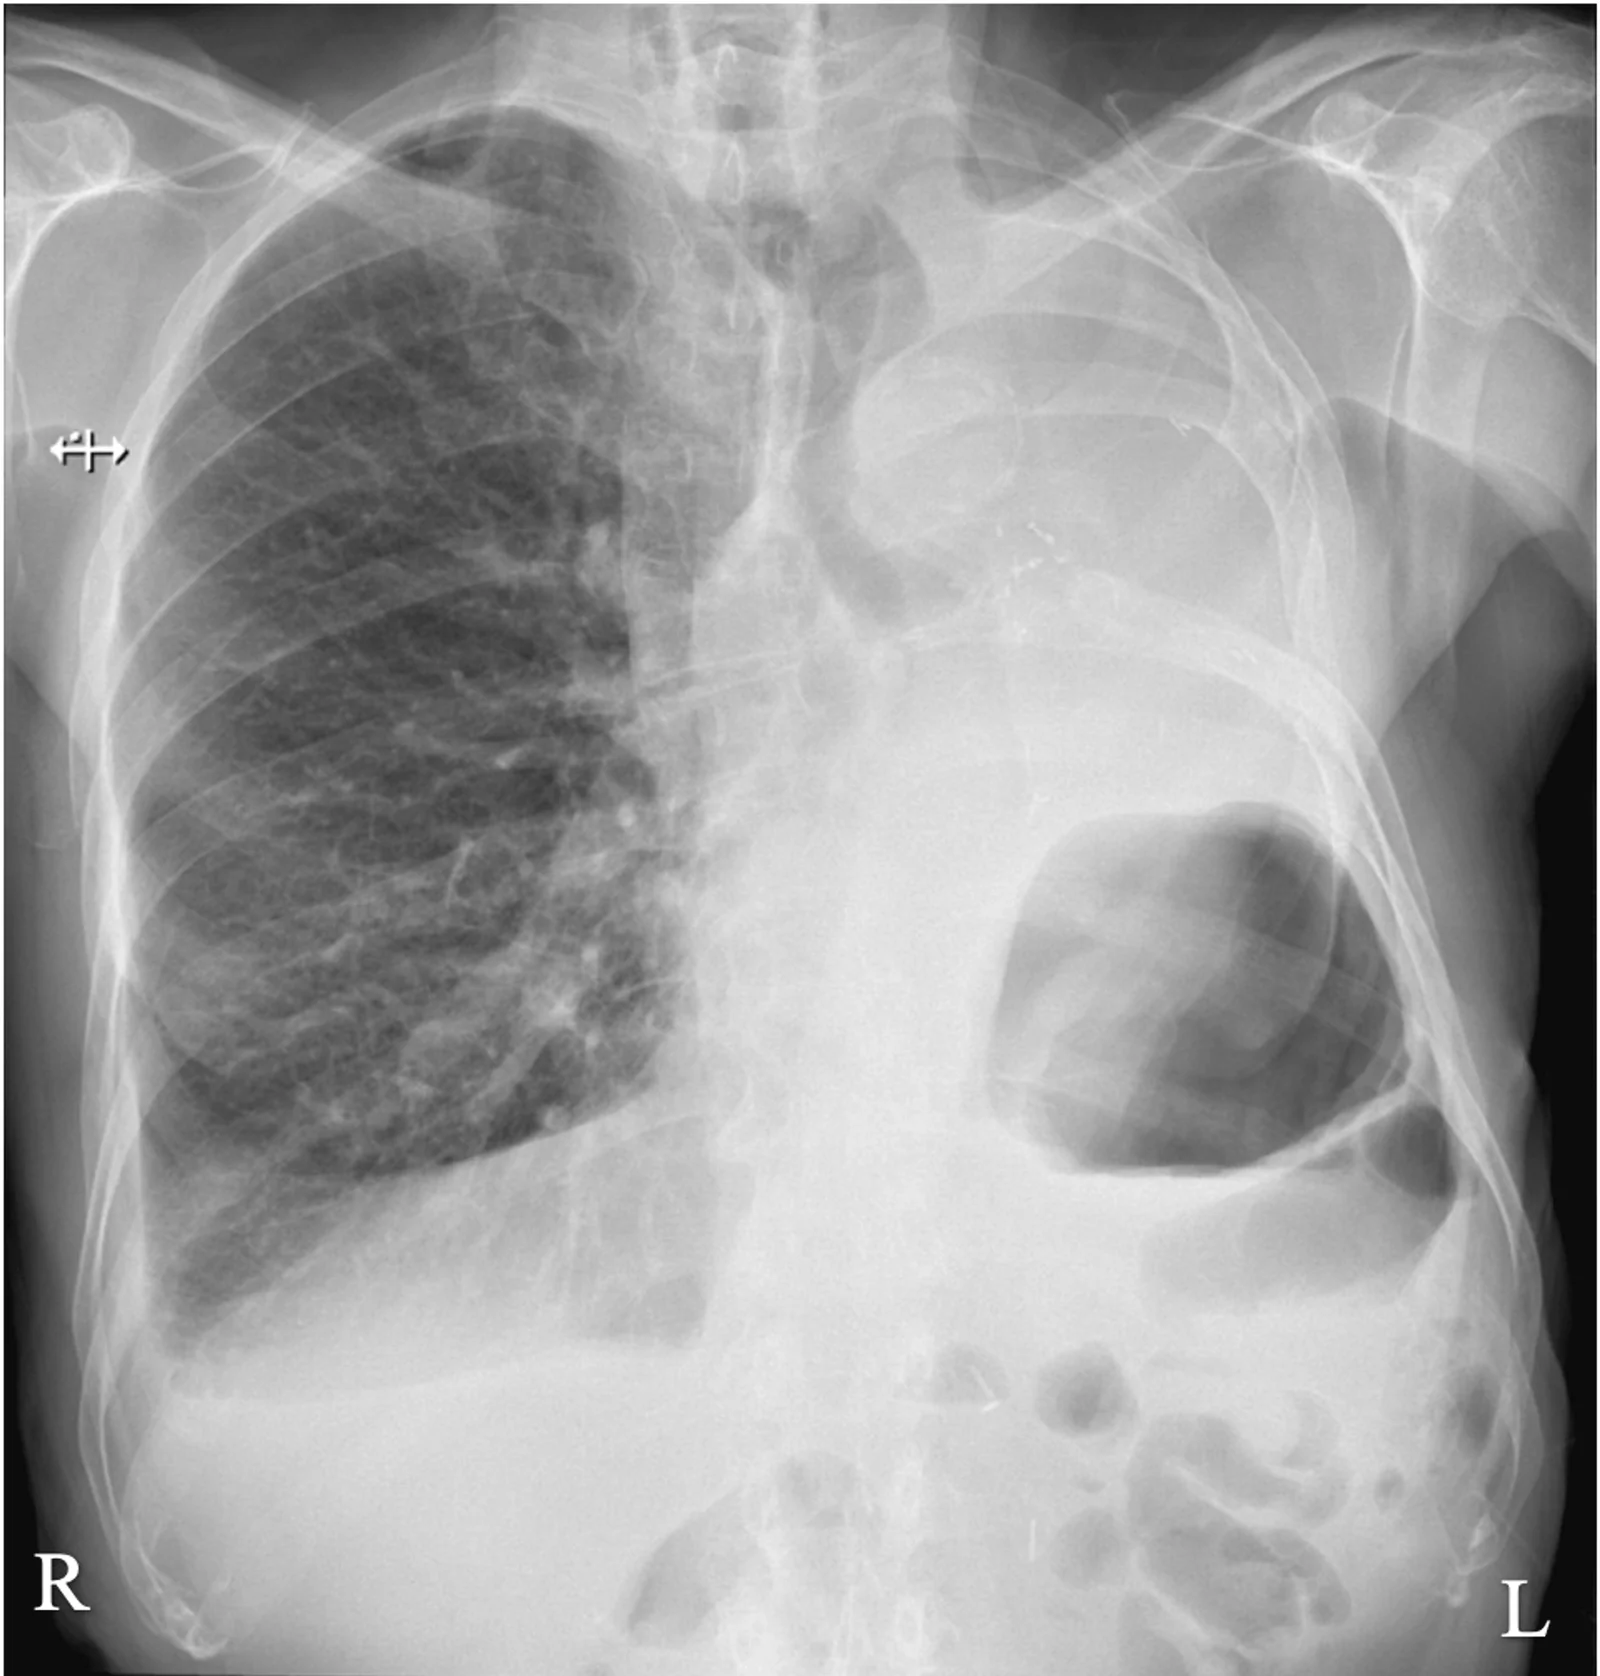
Postoperative chest radiograph after chest drain removal Chest radiograph obtained on postoperative day 6 demonstrating complete re-expansion of the right lung and absence of recurrent pneumothorax. L: left; R: right
